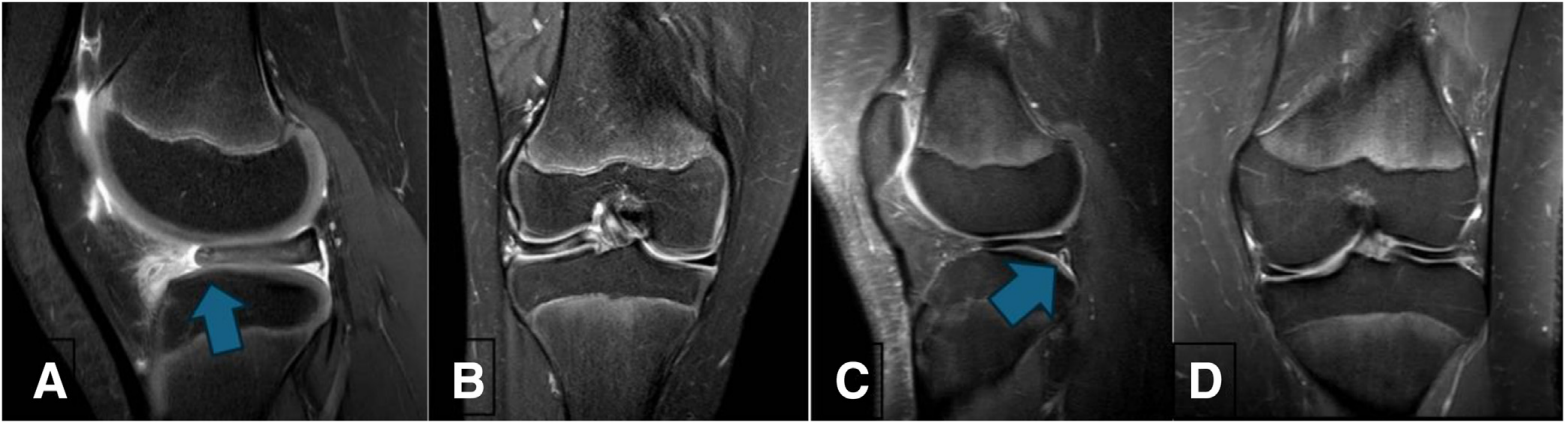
Representative sagittal (A) and coronal (B) cuts of proton-dense fat-suppressed sequences of a right knee with anterior peripheral rim instability (PRI) patient showing posterior displacement of the anterior horn of the lateral meniscus (arrow). There is resultant signal hyperintensity remains where the anterior horn should be attached. The coronal image shows the lack of visible lateral meniscus where it should be present compared with the contralateral side. An example of a posteriorly located fluid collection (arrow) in (C) and (D) is suggestive of isolated posterior instability for a patient with nonanterior PRI. All magnetic resonance imaging scans were obtained in the supine position with the knee extended.
